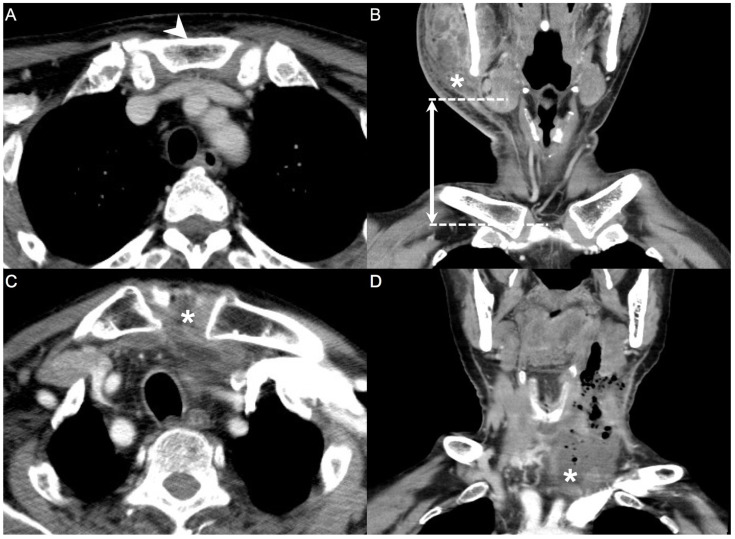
Computed tomography (CT) parameters. Distance measured from the level of the abscess to the level of the sternal notch (A,B). Downward invasion of the abscess to the sternal notch, leading to mediastinitis (C,D). Arrowhead: sternal notch; asterisk (*): deep neck abscess; dotted line: horizontal level; double arrow: distance between the level of the abscess and the level of the sternal notch in CT (300 × 300 dpi).
